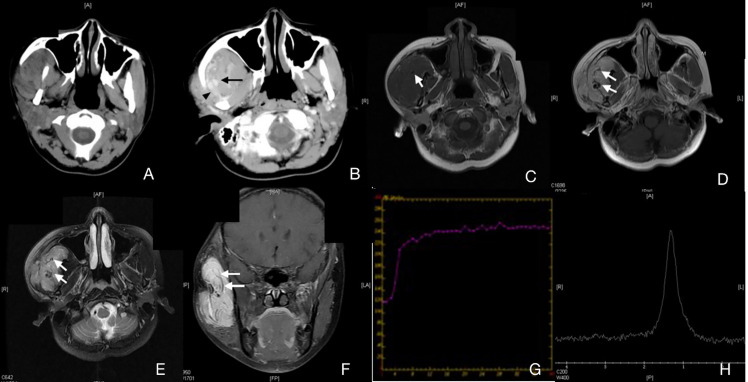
Case 4 (Supplementary Table 1), capillary IMHs. (A) Axial CT image showing an enlarged right masseter with uneven attenuation. (B) Axial enhanced CT image showing an apparently enhanced vermicular vessel (arrow) and an enlarged regurgitant vein (arrowhead). (C) Axial T1WI showing a lesion with an intermediate signal intensity and a vague border. (D) Axial T2WI showing a lesion with a slightly high signal intensity and a blurred border. (E) Axial enhanced fat suppressed T1WI showing an unevenly enhanced lesion in the right masseter. (F) Coronal enhanced fat suppressed T1WI showing a diffuse, irregular lesion with low signal intensity and a striped structure. This structure can also be found in panels (C–E). (G) TIC analysis showed quick wash-in and slow washout. (H) MRS showing no Cho peak at 3.2 ppm.
